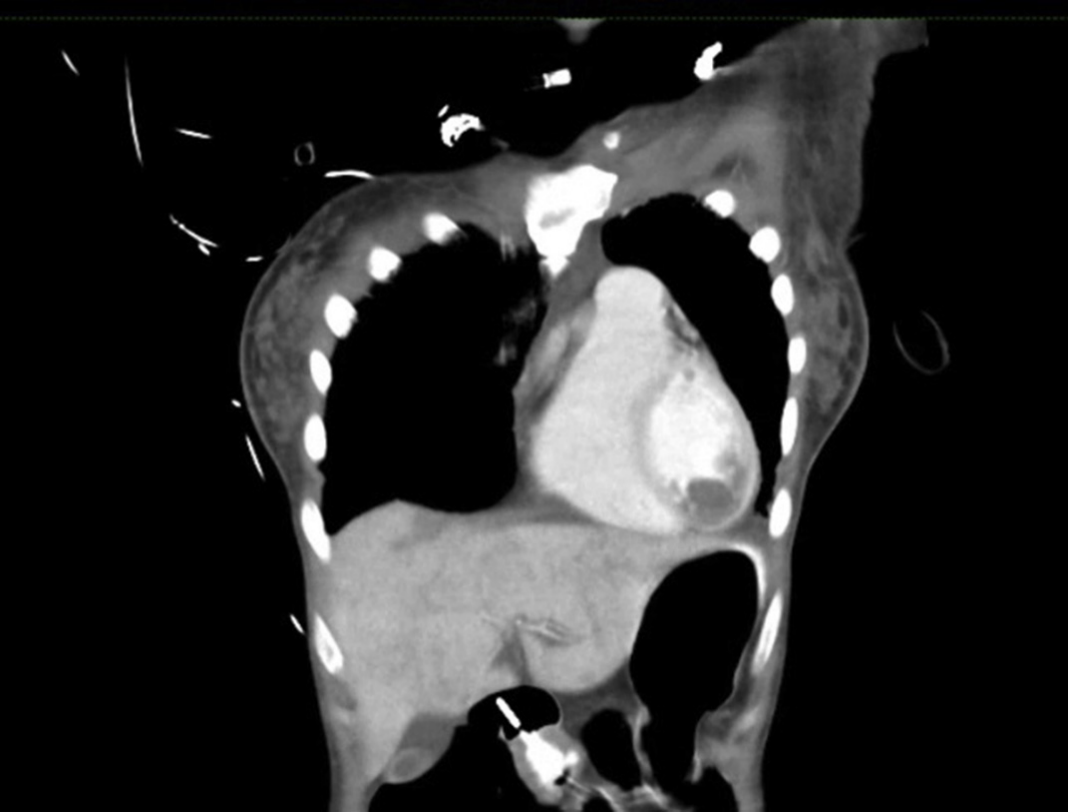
Chest Computed Tomography Showing Coronal View of the Left Ventricular Apical Thrombus
